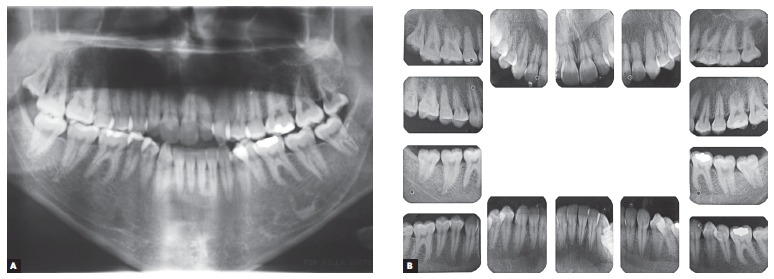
Initial panoramic (A) and periapical (B) photographs.
